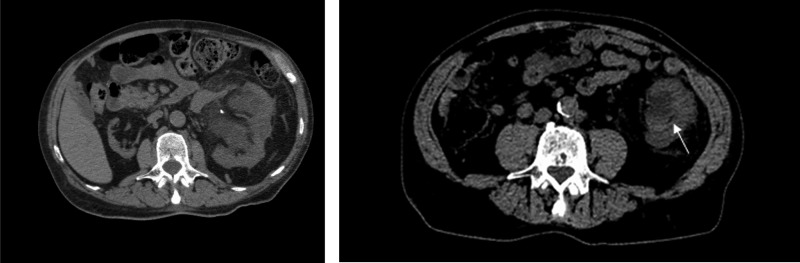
Noncontrast CT scan images. *Left panel* showing severe hydronephrosis of left kidney with a vascular calcification and an atrophic right kidney. *Right panel* showing necrotic debris in renal collecting system (*arrow*).
